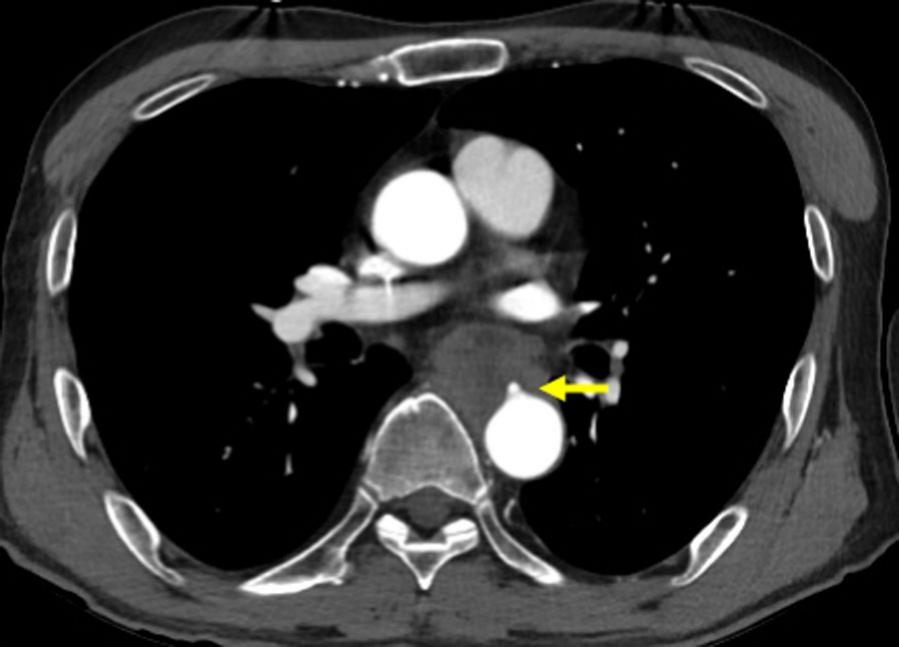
Computed tomography at the onset of aortoesophageal fistula showed extravasation into the esophagus from the thoracic descending aorta (arrow)
